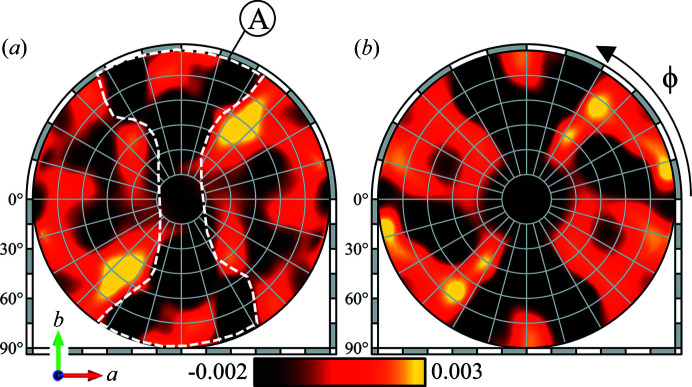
Mn *K*α hologram obtained at (*a*) a high incident X-ray energy of 6.565 keV (*χ*
